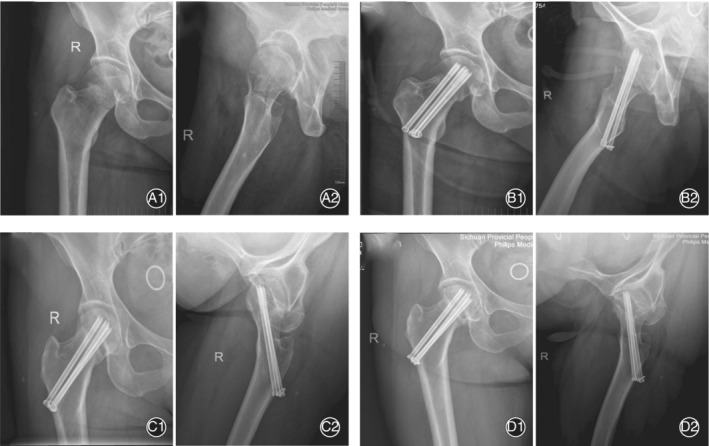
A 53‐year‐old man who had necrosis of the femoral head, after internal fixation with three cannulated screws using the traditional procedure. (A) Preoperative anteroposterior (AP) and lateral hip X‐ray images. (B) Postoperative X‐ray images. (C) 18 months after the operation, the fracture healed. (D) 28 months after the operation, necrosis and collapse of the femoral head could be seen on the radiographs.
